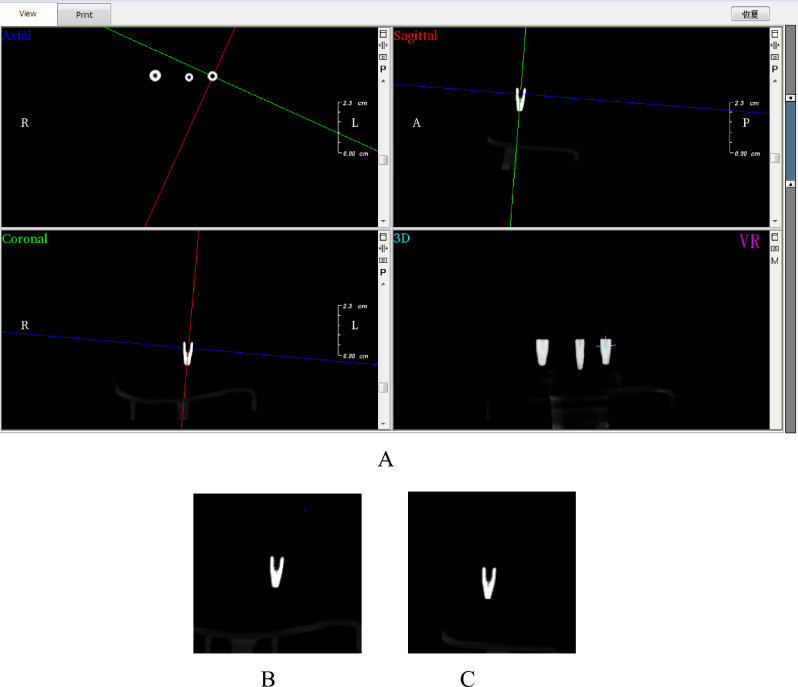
Screenshot window of CBCT. Note: (A) Screenshot window of CBCT with fixed Straumann 4.8–10 mm implant centric long axis unchanged. (B) Images in two dimensions created from coronal screenshots. (C) Images in two dimensions created from sagittal screenshots.
